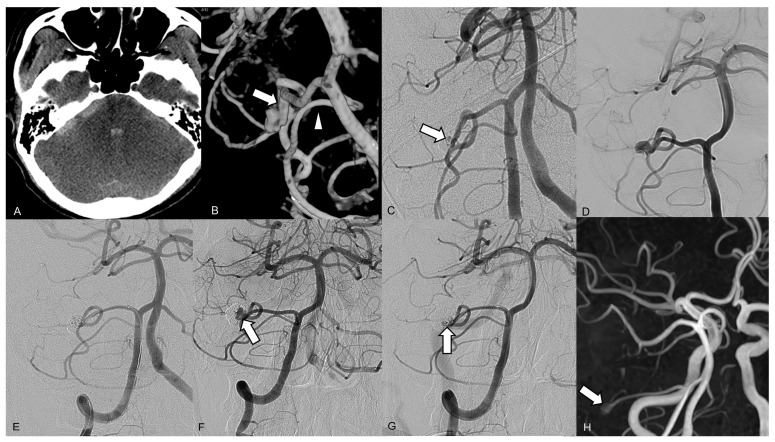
Brain images from a 42-year-old male who presented with a sudden onset of mental deepening. (A) The axial brain computed tomography scan on admission shows a subarachnoid hemorrhage, predominantly distributed in the right cerebellopontine angle cistern, and a small intraventricular hemorrhage. (B) A three-dimensional reconstruction of the right vertebral artery angiogram reveals a lobulated wide-neck aneurysm arising from the right distal anterior inferior cerebellar artery (AICA) within a meatal segment. The right AICA (arrow) was larger than the posterior inferior cerebellar artery (arrowhead). (C) The right vertebral artery angiogram performed immediately after the endosaccular embolization shows a tiny remnant portion of the neck (arrow). (D) The 3-month follow-up angiograph shows major recanalization at the superior part of the coiled aneurysm. (E) The final angiogram after the second coil embolization shows complete occlusion of the pseudoaneurysm, with preserved distal AICA flow. (F) The 2-year follow-up angiograph shows major recanalization (arrow) at the base of the coiled pseudoaneurysm. (G) The final angiogram after the third coil embolization shows near complete occlusion of the pseudoaneurysm, with a tiny remnant lesion (arrow) at the base. (H) Follow-up magnetic resonance angiography, 4 years after the final embolization, shows a small remnant sac (arrow) at the base of the coiled pseudoaneurysm.
